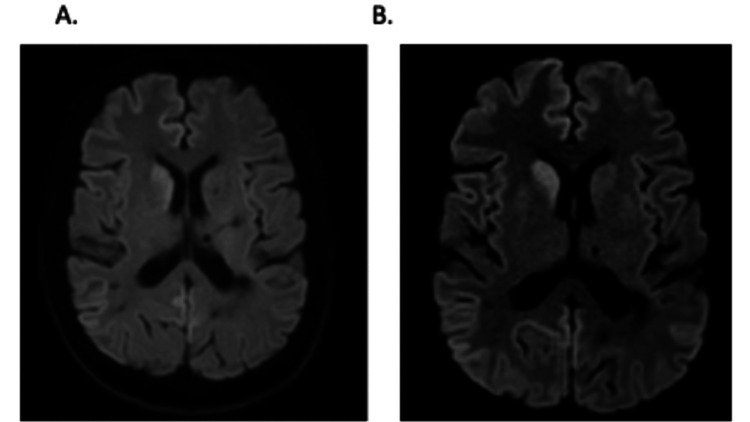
MRI of the patient's brain A: Initial brain MRI of the patient one month prior to presentation B: The post-admission diffusion-weighted image and T2-FLAIR brain MRI shows cortical diffusion abnormality asymmetrically involving the right cerebral hemisphere, basal ganglia diffusion abnormality with asymmetric involvement of the right caudate head and anterior lentiform nucleus, cortical ribboning, and confluent supratentorial and pontine white matter hyperintensity. FLAIR: Fluid-attenuated inversion recovery
